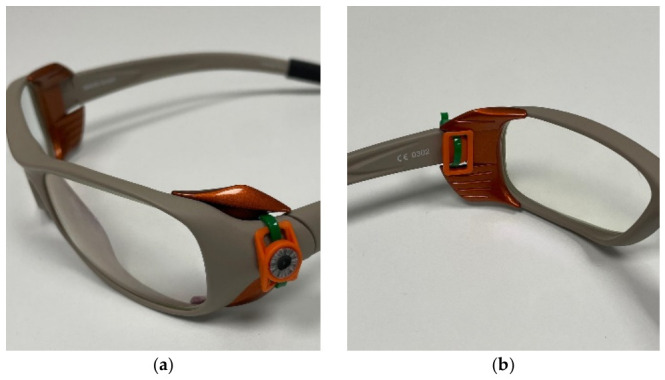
Thermoluminescent Dosimeter fixed to the outer (a) and inner (b) frames of the X-ray protective glasses to measure the unprotected and protected ocular doses.
